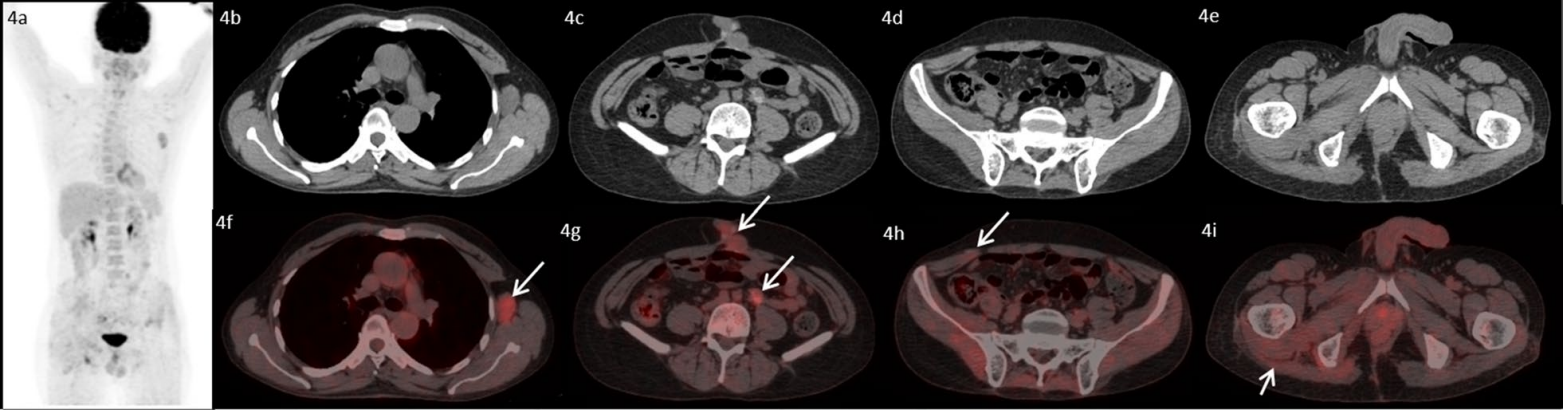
18F-FDG PET/CT images of the patient six months after initiation of therapy with erlotinib. MIP image (4a) transaxial CT images (4b–4e) and fused PET/C images (4f–4i) show no significant interval change in size and metabolic activity of the left axillary lymph node, anterior abdominal wall lesion and omental/mesenteric deposits and lesion near left ischial tuberosity (arrows), suggestive of stable disease
